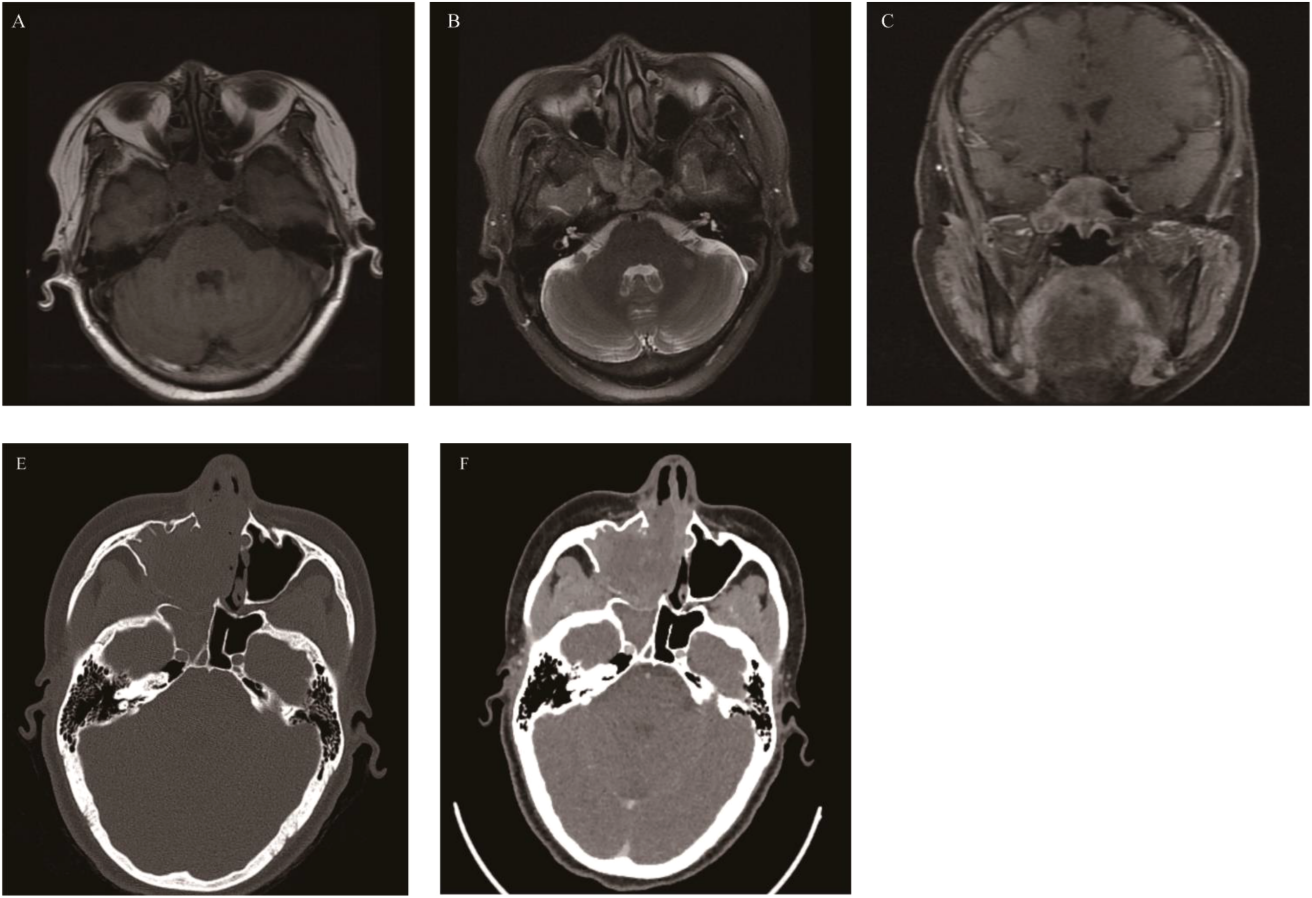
Olfactory neuroblastoma: Patient 1: An irregular mass was found in the sphenoid sinus, presenting as hypointense on T1WI, hyperintense on T2WI, and showing moderate enhancement on MRI contrast (A-C). Patient 2: Axial CT showed a soft-tissue density mass in the right maxillary sinus and sphenoid sinus with obvious bone destruction, and heterogeneous enhancement on CT contrast (E, F). The locations of the masses in both patients were atypical.
